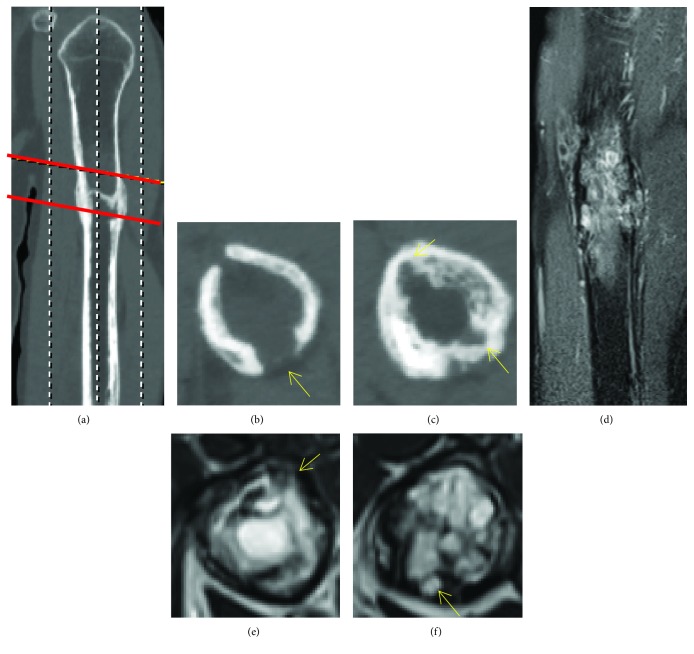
(a) Computed tomography of the left humerus showing ((b) upper line level of sagittal view) partial cortical destruction (yellow arrow) and ((c) lower line level of sagittal view) erosion (yellow arrow). (d) T2-weighted fat suppression magnetic resonance image (MRI) of the left humerus showing longitudinal invasion of the tumor, a nearly 7 cm extent in the diaphysis. Axial view of T2-weighted fat suppression of MRI revealing tumor invasion to the (e) extracortical lesion (yellow arrow) and (f) intracortical lesion (yellow arrow).
